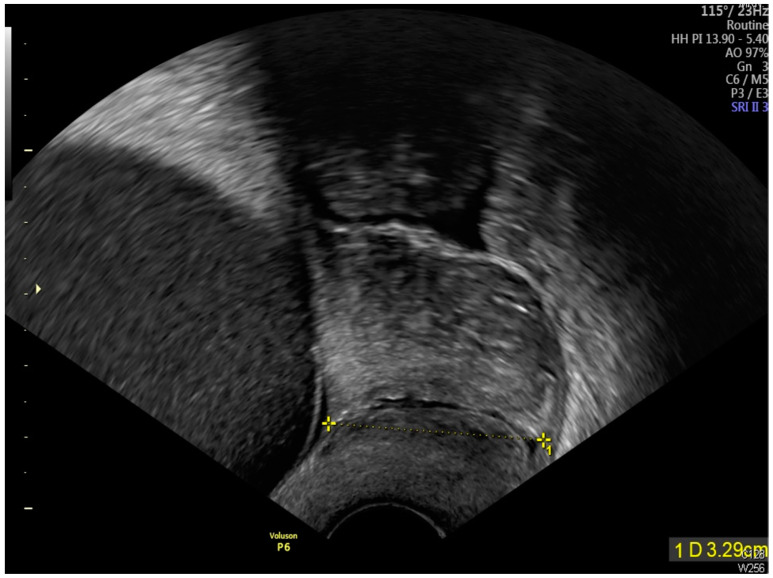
Transvaginal sonographic assessment demonstrated a cervical length measurement of 3.29 cm (As shown by the dotted line distance), with no evidence of cervical dilation.
